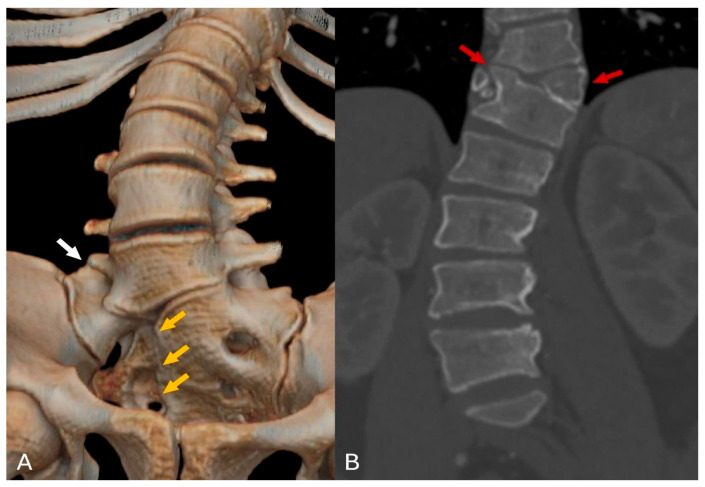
CT reconstruction and coronal CT imaging: (A) The 3D CT reconstruction reveals defects in the sacral vertebrae on the right side, indicative of type IVB hemisacrum (orange arrows), along with sacralization of the L5 vertebra on the right side, categorized as Castellvi classification IIIA (white arrow). (B) The coronal CT image displays a butterfly deformity at the Th11 level, accompanied by fusion of the Th11 and Th12 vertebrae on the right side (red arrows).
